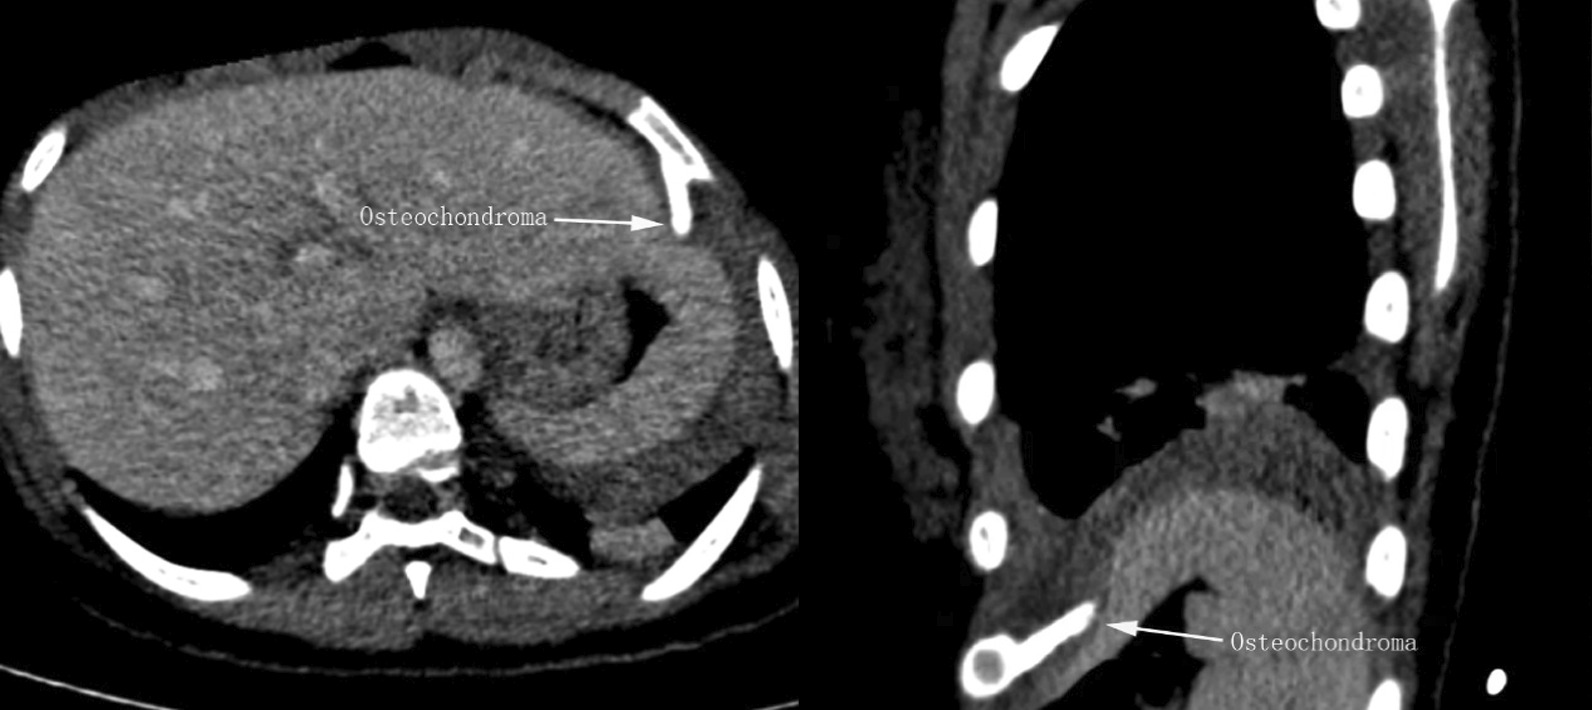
(left), (right): Obliquely axial and sagittal contrast-enhanced CT showing focal impression of hepatic left lobe from the bony lesion, indicative of abutting the diaphragm
